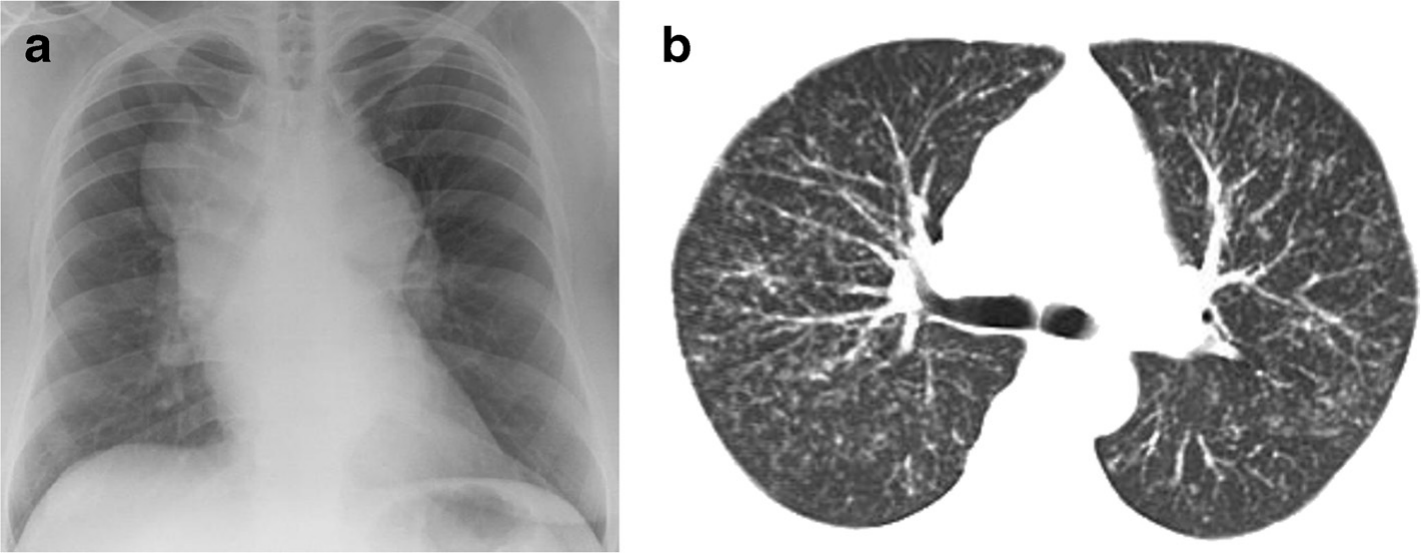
a Chest radiograph showing massive hilar and mediastinal lymphadenopathy and b, thoracic CT scan showing diffuse nodular infiltration of the lung interstitium. Neither of these patients complained of cough.
